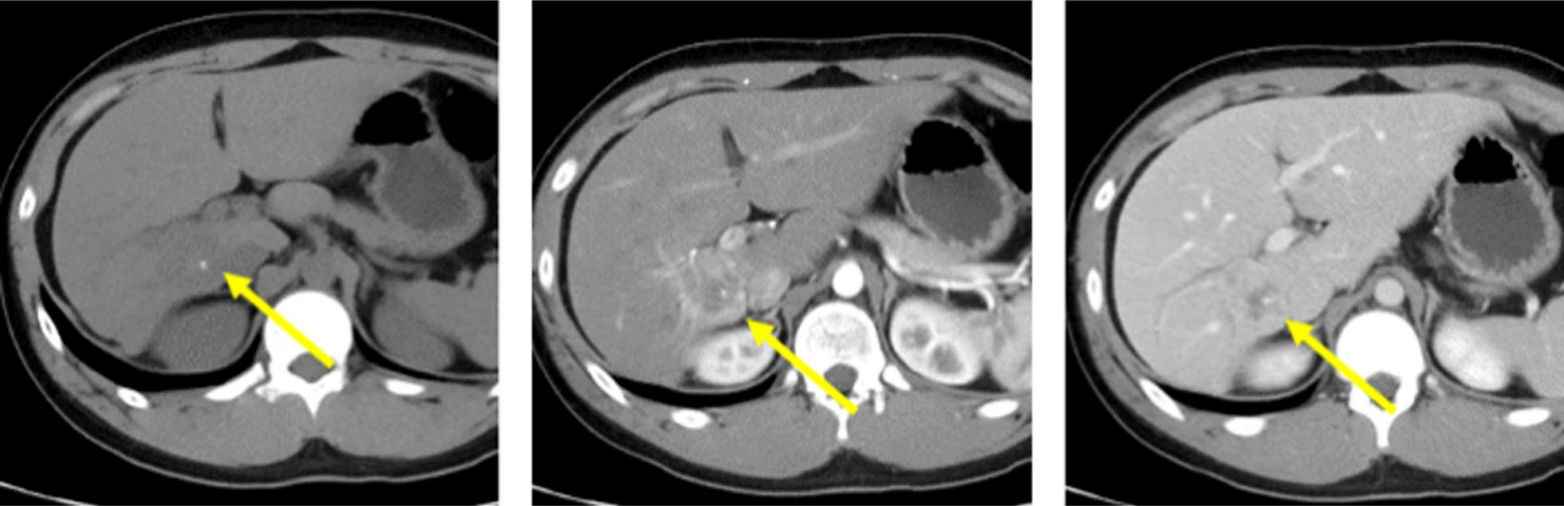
Abdominal CT showing a 35-mm solid tumor, which contrasts at the early phase of dynamic enhanced study of the hepatic segments 6, 7, and 8, with occlusion of the right portal vein and calcification in the tumor
